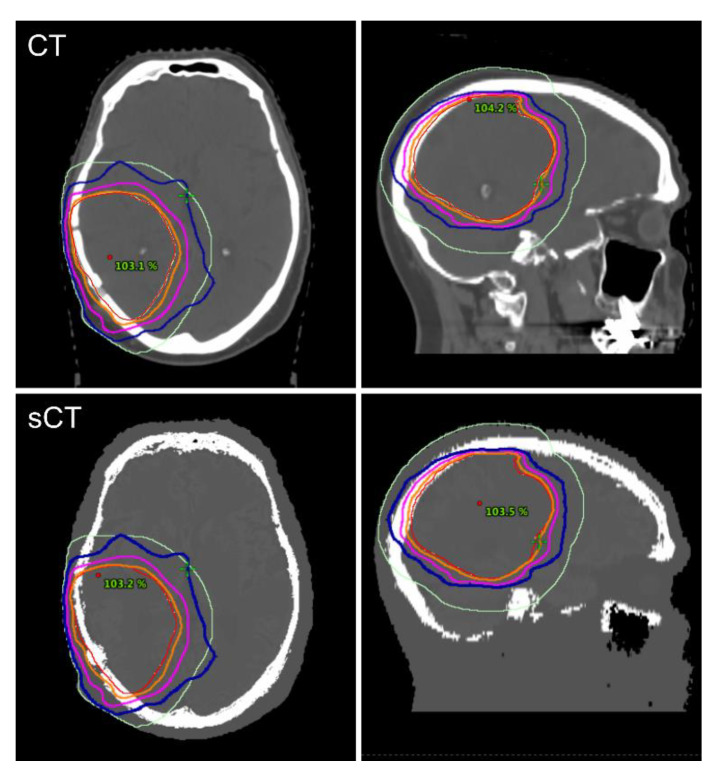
Case example of the RTP comparison between CT- and sCT-based RT plans of a glioma patient. Isodose levels of 90% (orange), 70% (magenta), and 50% (blue) are visualized together with PTV (red) and OAR (light green) contours in transversal and sagittal planes (RTP: radiotherapy treatment planning, CT: computed tomography, sCT: synthetic computed tomography, RT: radiotherapy, PTV: planning target volume, OAR: organs at risk).
